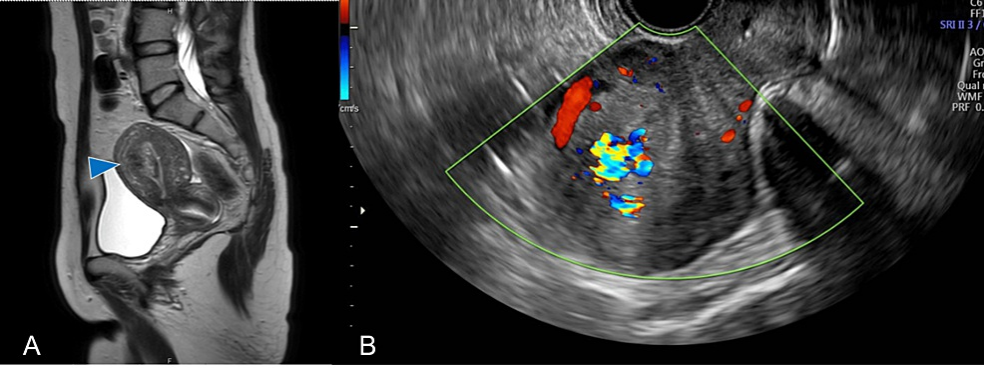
MRI and ultrasound for the diagnosis of RPOC A: Magnetic resonance imaging (MRI) showing a mass measuring 20×16×27 mm in size (arrows) protruding into the front wall of the endometrial cavity on a sagittal T2-weighted image. B: RPOC with hypervascularity in the intrauterine region. MRI: magnetic resonance imaging, RPOC: retained products of conception
